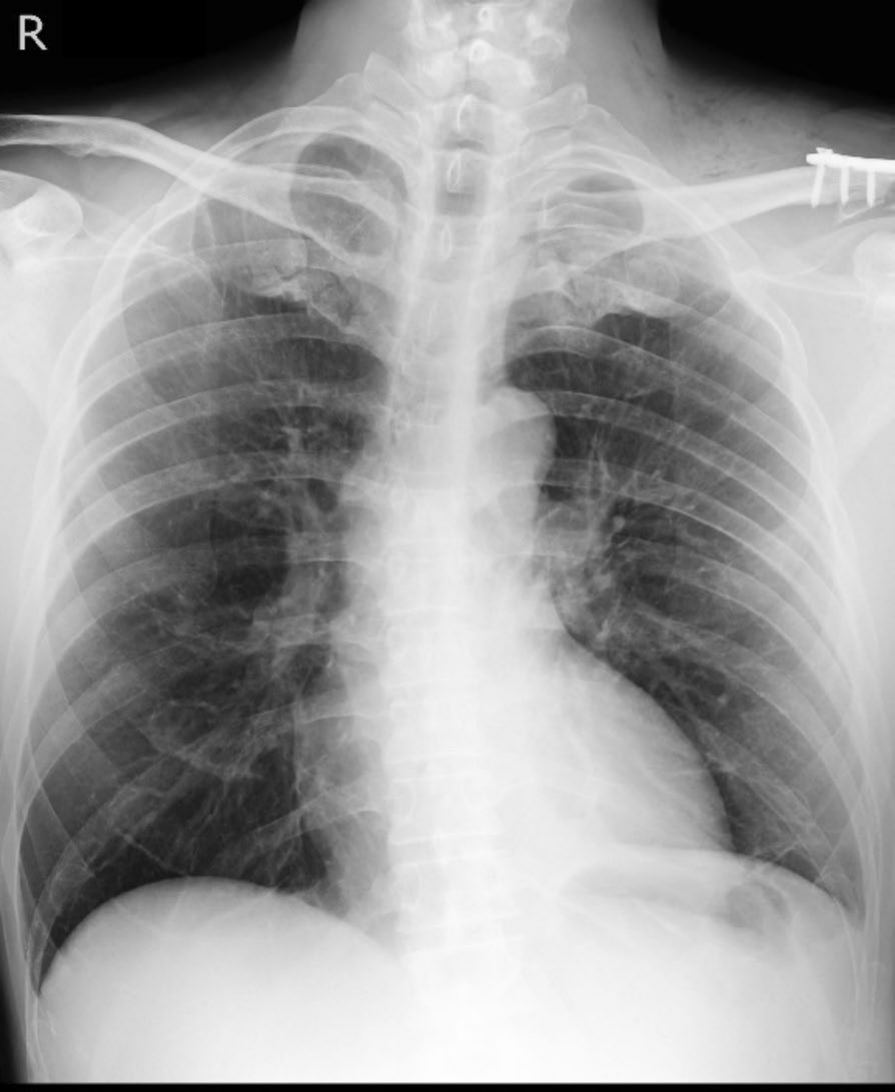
Chest radiography on postoperative day 1 showing further improvement of left lung inflation
